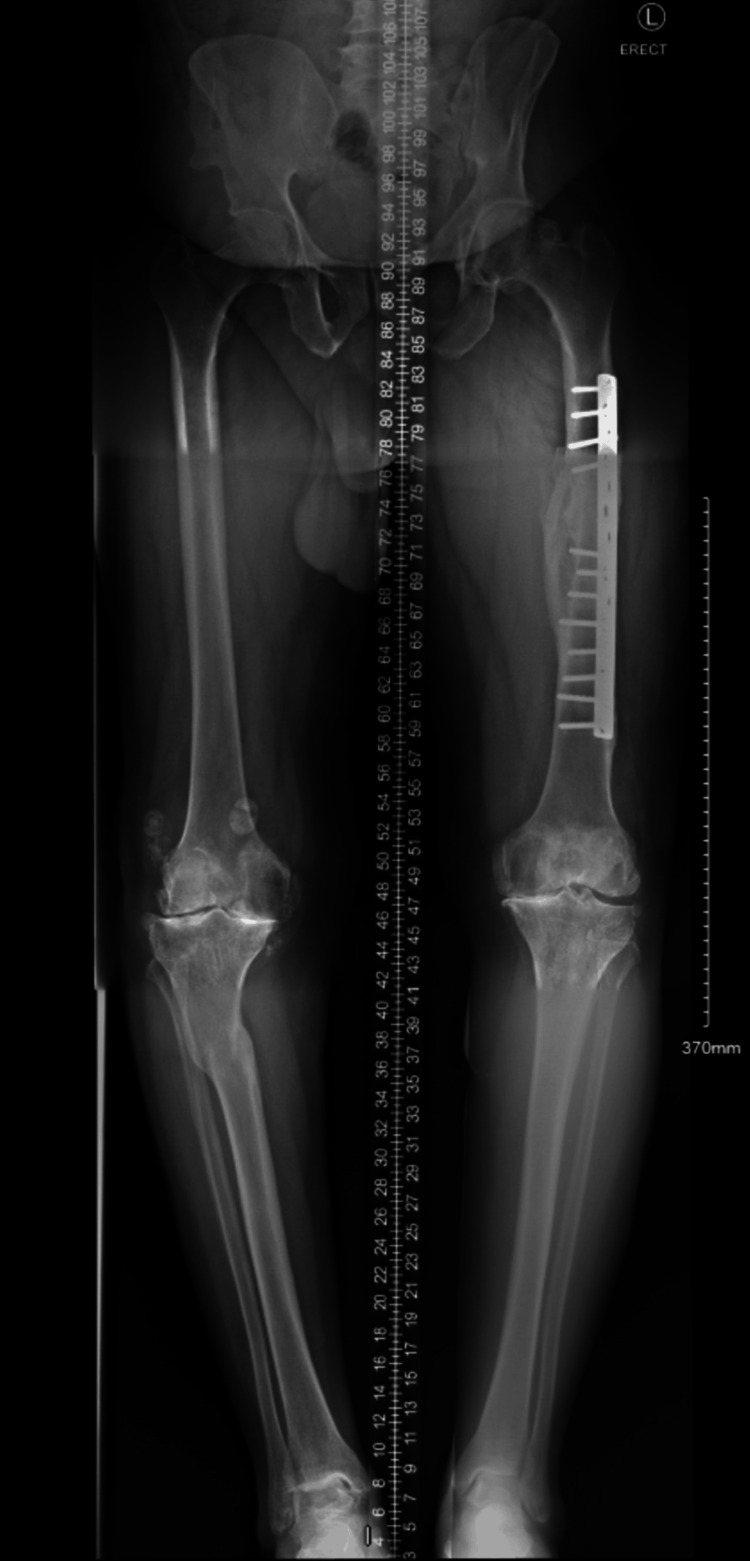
Full-length standing radiography showing right-sided post-traumatic tibia varus deformity of 17°
